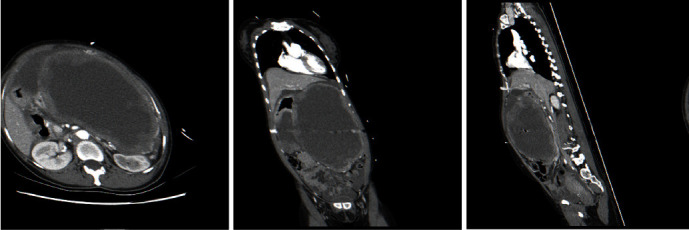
CT of the chest/abdomen/pelvis with contrast showed a large predominantly cystic lesion with its epicenter in the peritoneal cavity, its wall has nodular thickening and heterogenous peripheral enhancement with central cystic/necrotic area. Free fluid (25HU) was also appreciated in the abdomen and pelvis. A significant mass effect was seen on the retroperitoneal structures as well as the stomach and bowel loops. The mass measures 21 cm × 15 cm × 24 cm and is in direct contact with the greater curvature of the stomach.
